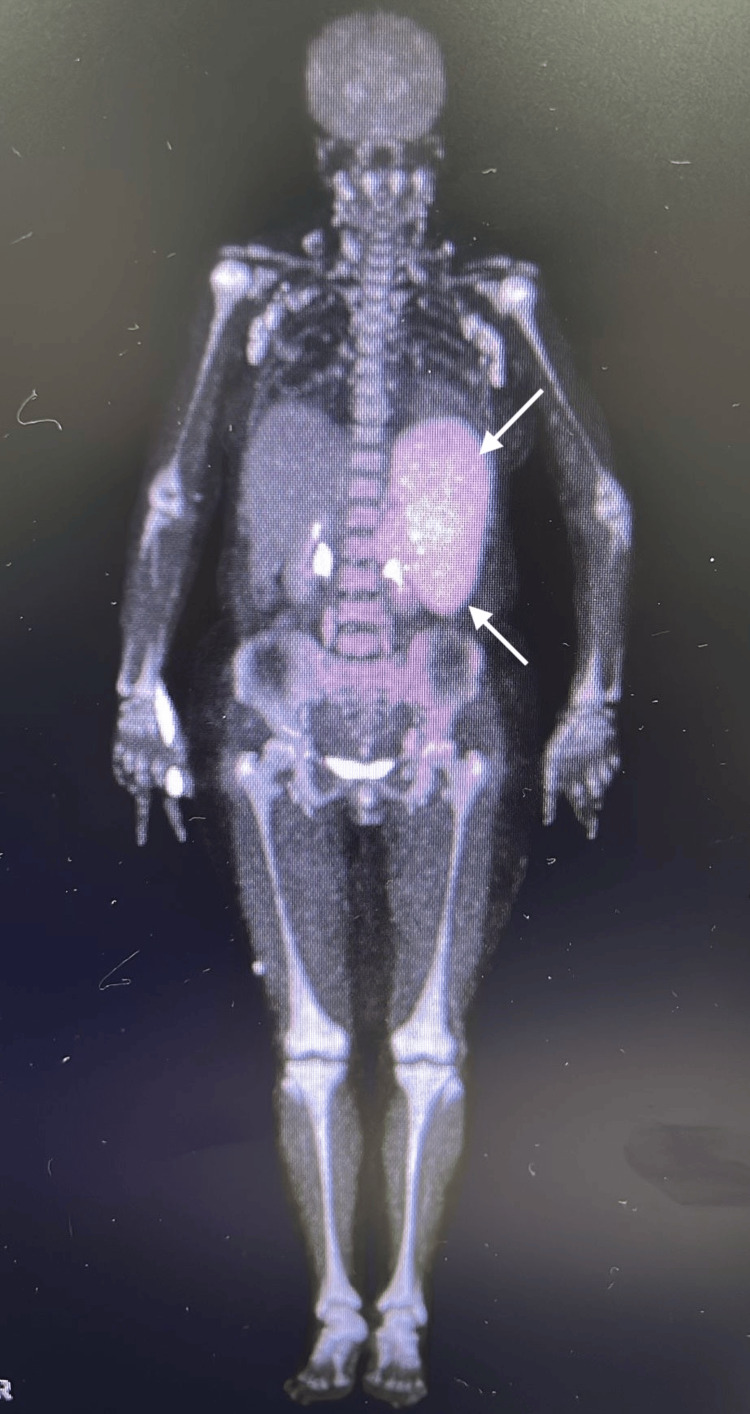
PET-CT showing splenomegaly. PET-CT: positron emission tomography-computed tomography
